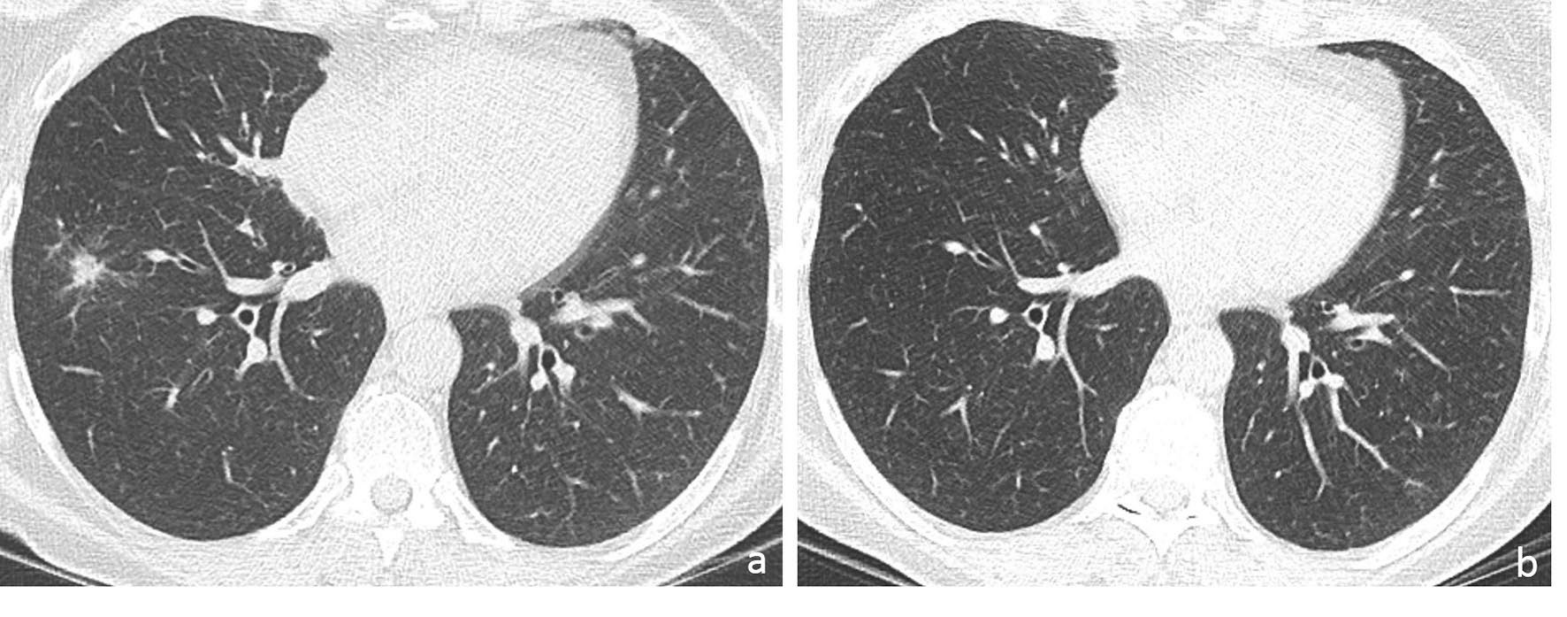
Axial CT image (a) shows a solid nodule with halo sign in the right lower lobe that was categorized as CO-RADS 2 for its typical appearance for other infectious disease (likely bacterial), as demonstrated by its complete disappearance after antibiotic therapy (b)
